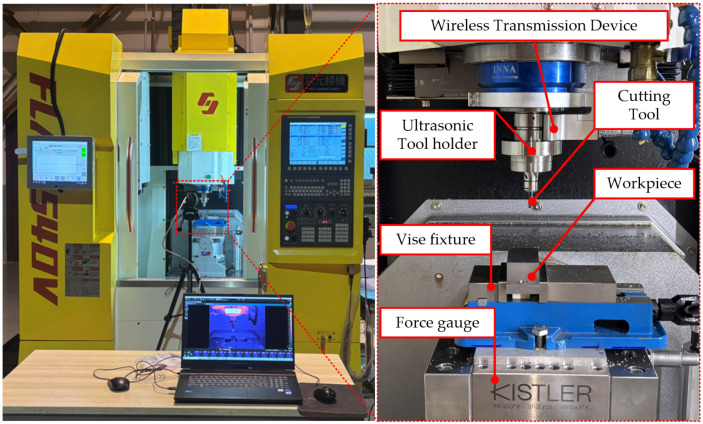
Experimental equipment of ultrasonic vibration milling.
